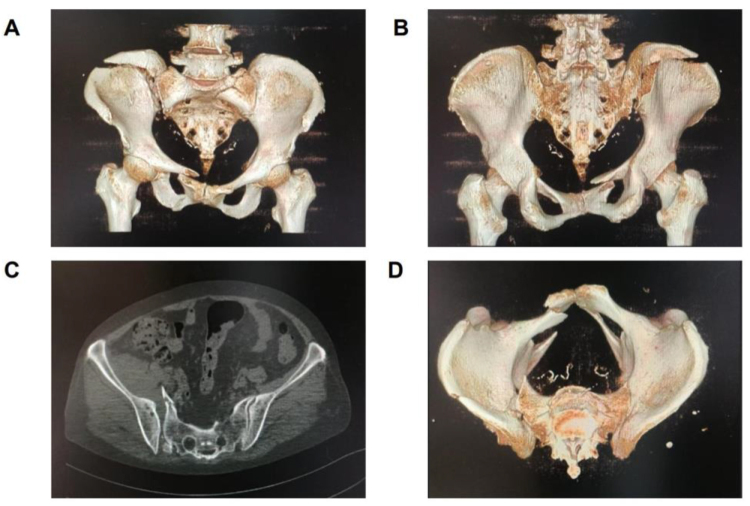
Preoperative CT results of the patient. (A) Anterior view of CT reconstruction of pelvis. (B) Posterior view of CT 3D reconstruction of pelvis. (C) CT tomography of sacroiliac joint separation. (D) Top view of CT reconstruction of pelvis.
